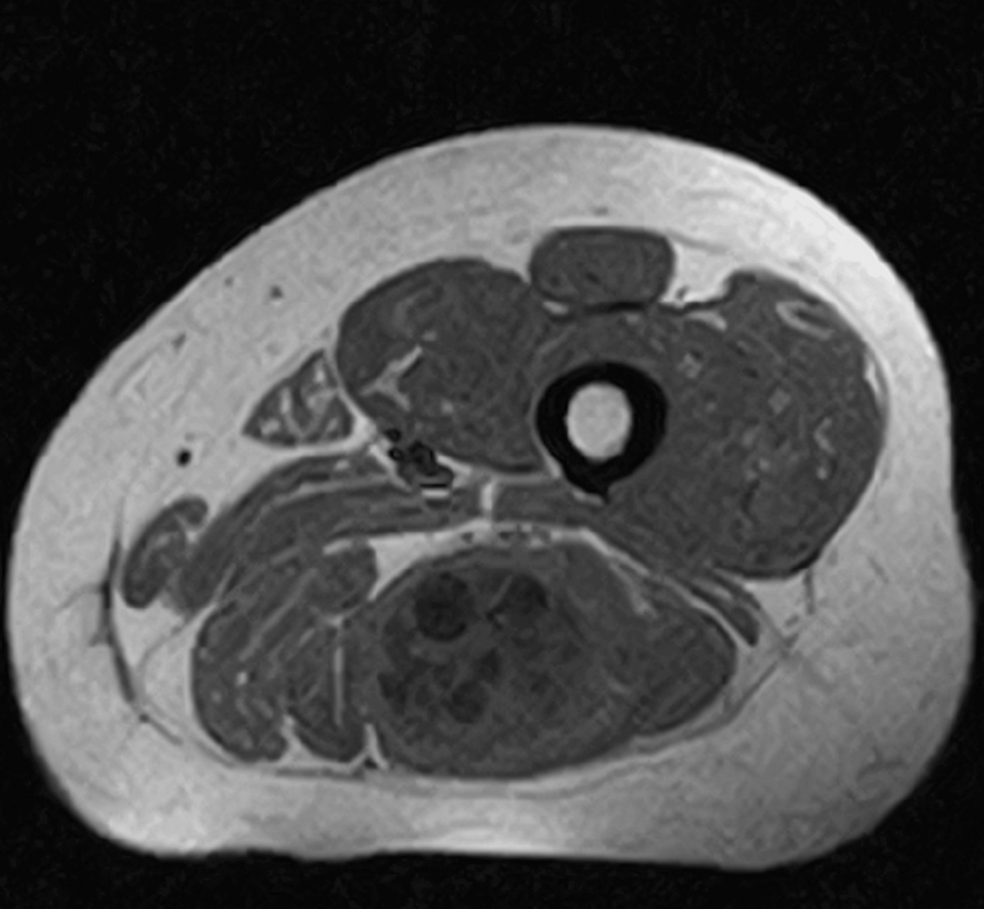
Cross-sectional MRI demonstrating the lesion in the left thigh
